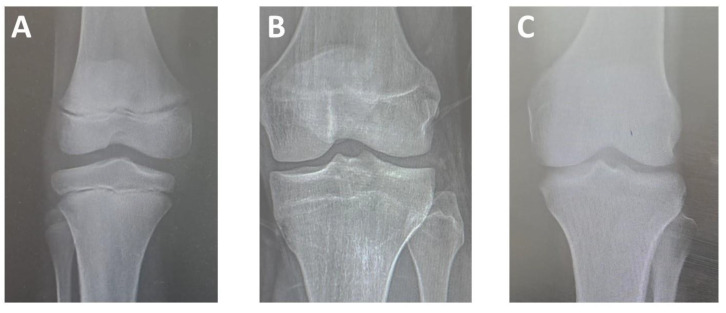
Plain X-ray images of the knee joint showing stages of epiphysis union; (A) Stage I, epiphysis is not fused at the distal end of the femur and the proximal ends of the fibula and tibia; (B) Stage II: epiphysis is fully ossified, and epiphyseal scar is visible at the distal end of the femur and the proximal end of the tibia; (C) Stage III, epiphysis is fully ossified, and epiphyseal scar is not visible at the distal end of the femur and the proximal end of the tibia [12].
